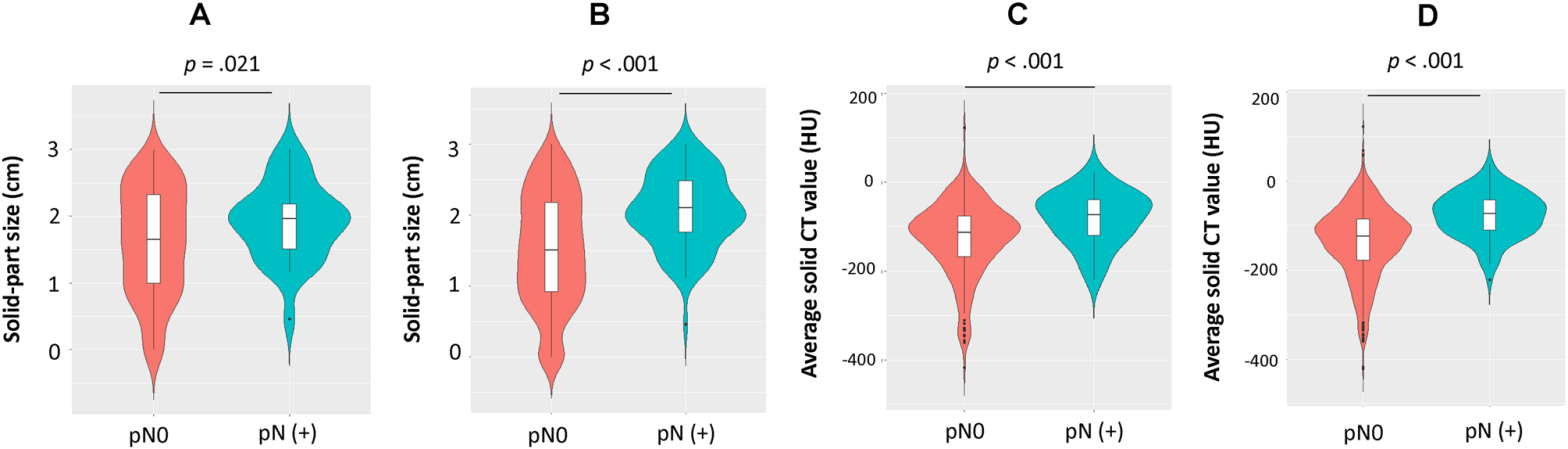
Violin plots for the comparison of the distribution of solid-part size in the validation cohort (A) and entire cohort (B), and average solid CT value in the artificial intelligence in the validation cohort (C) and entire cohort (D). pN( +)—pathological lymph node positive; CT—computed tomography; HU—Hounsfield units.
